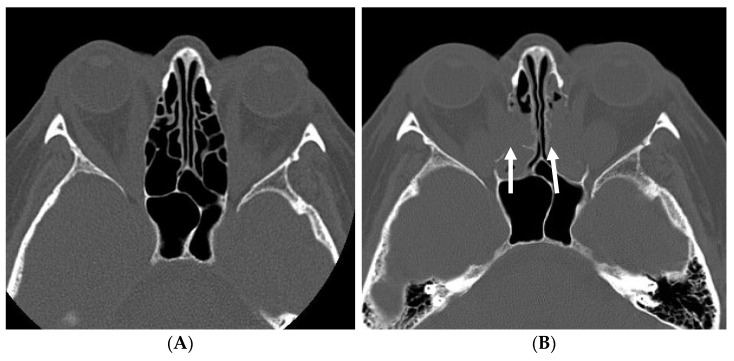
Axial CT scans showing orbital fat protruding into paranasal sinuses. Status before (A) and after (B) decompression. The fatty tissue filling the nasal cavity is visible (arrows).
